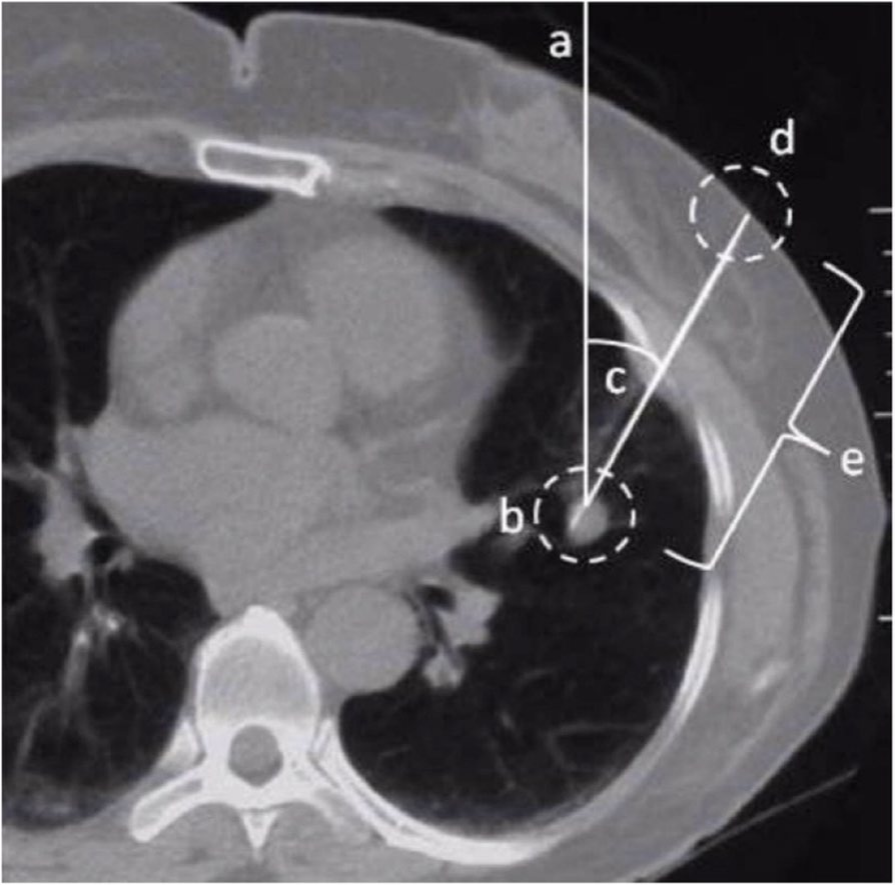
In the image a cross section of a human thorax is shown. a True vertical of the CT coordinate system, b target, c angle with respect to the vertical line, d entry point, e depth
